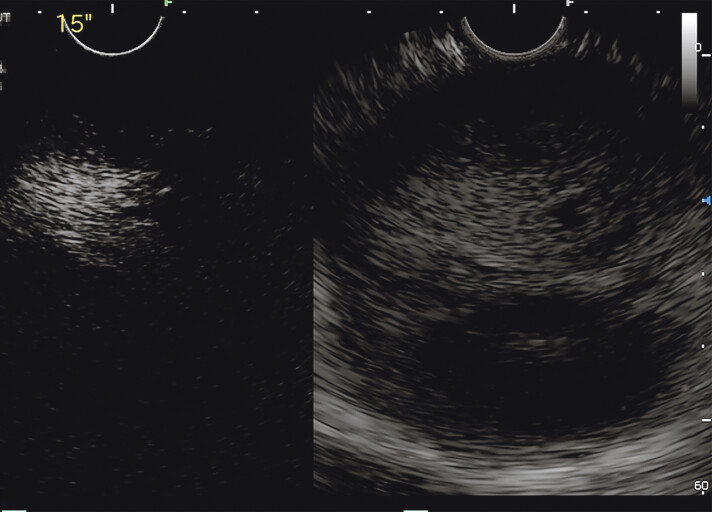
Contrast-enhanced endoscopic ultrasonography showed a heterogeneous hyper-enhancing pattern into the lesion.
